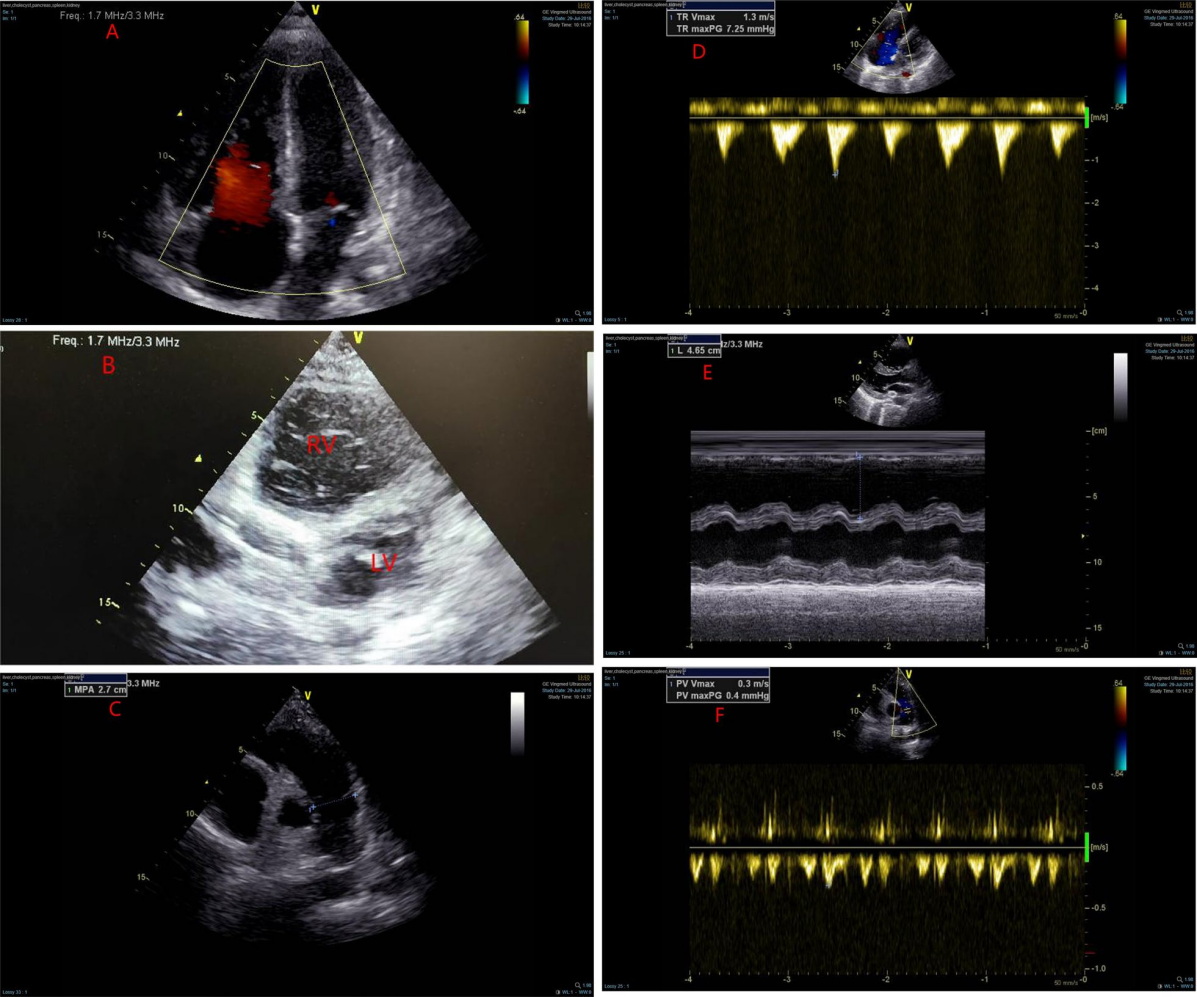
Cardiac Ultrasonographic image at presentation: Ultrasonographic image showing dilated right atrium and ventricle, severe regurgitated tricuspid valve and normal inner diameter of pulmonary artery. A, D Severe tricuspid valve regurgitation. B, E Marked right ventricular dilation. C, F Normal inner diameter of pulmonary artery
